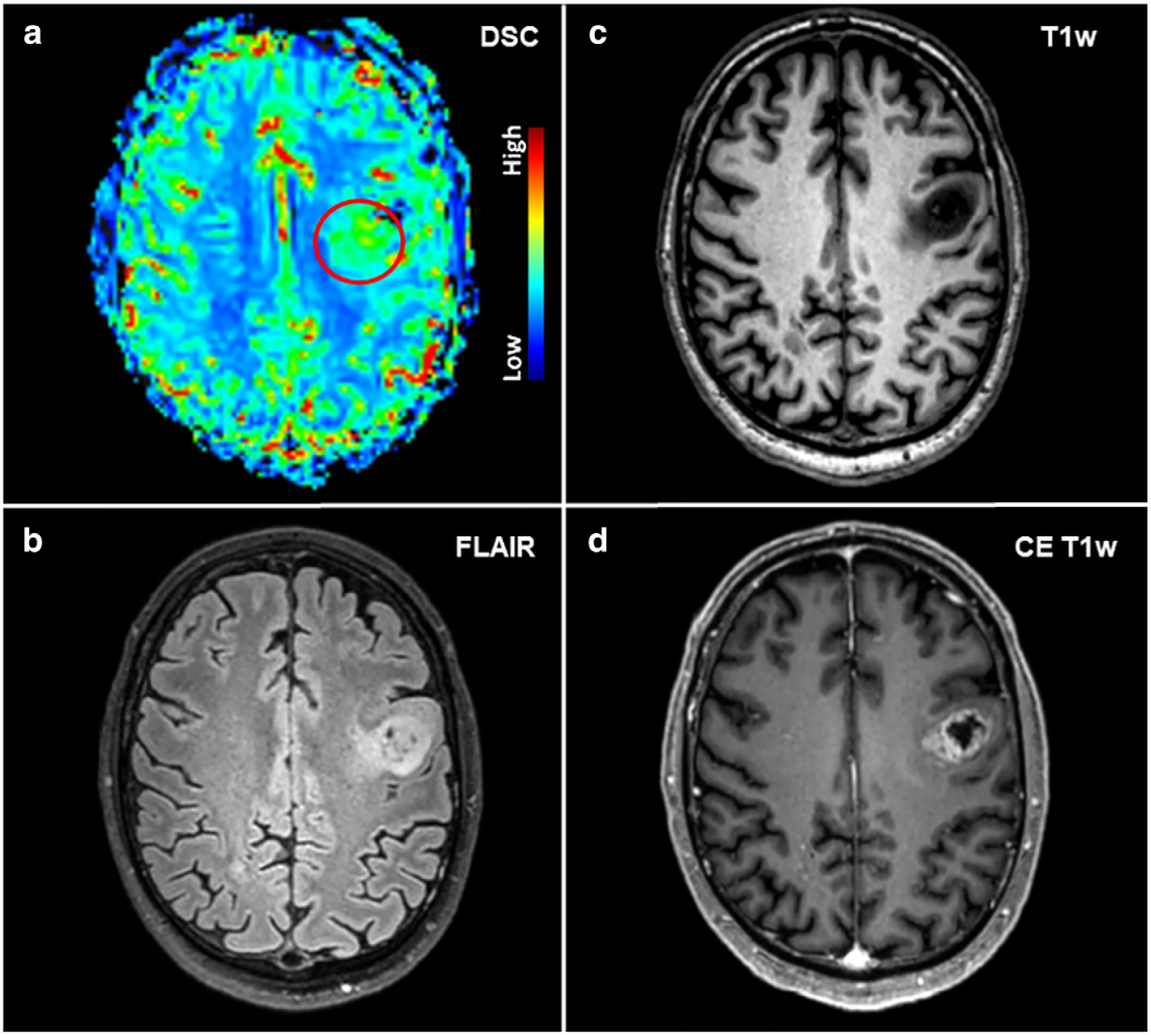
Elevated perfusion according to dynamic susceptibility contrast (DSC) MRI (a) in a 55‐year‐old male patient with a left frontal high‐grade glioma (HGG) that showed high signal on fluid‐attenuated inversion recovery (FLAIR); (b) imaging and contrast enhancement on T1‐weighted imaging (c, axial non‐contrast, and d, axial contrast‐enhanced images). The borders of the lesion with contrast‐enhancing tumor parts, in particular, showed hyperperfusion on DSC MRI (red circle, a).
